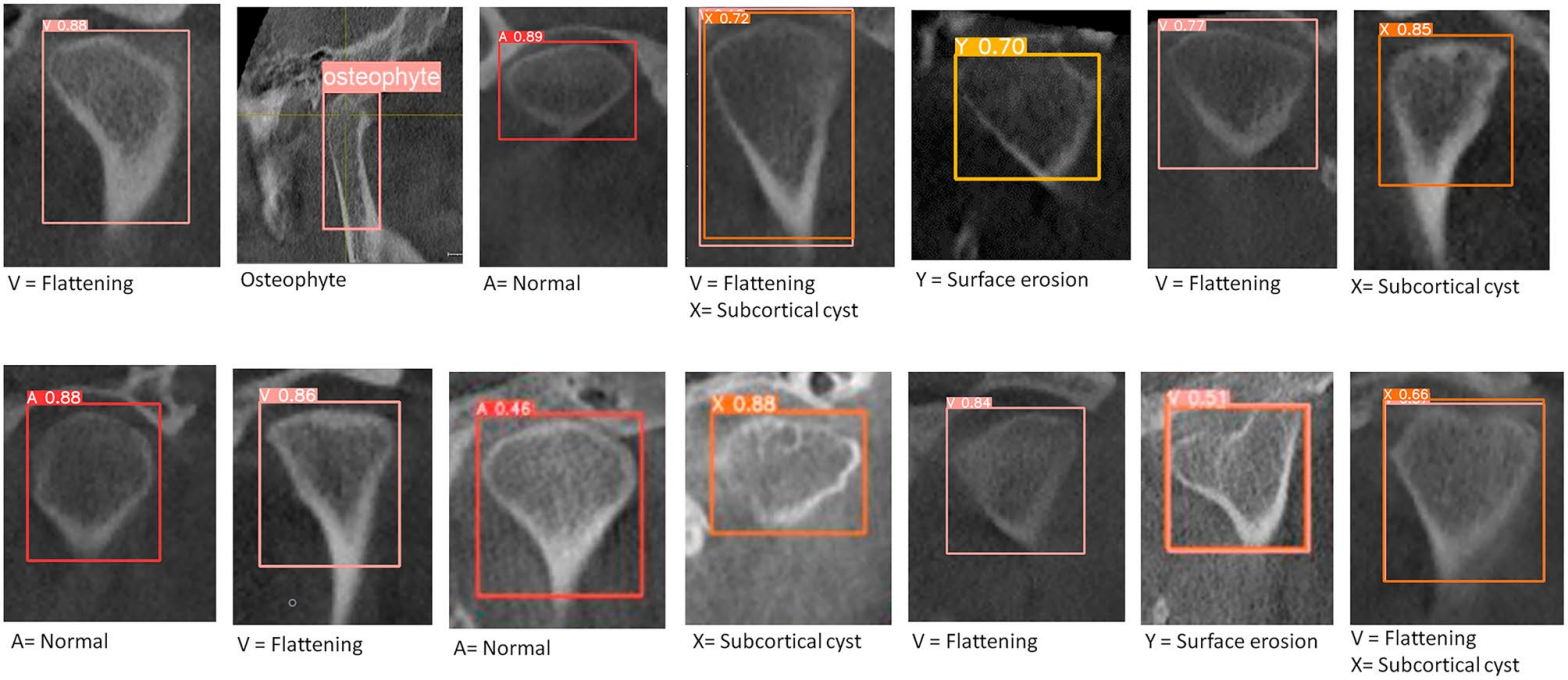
Sample CBCT images used in the validation of the AI model.
